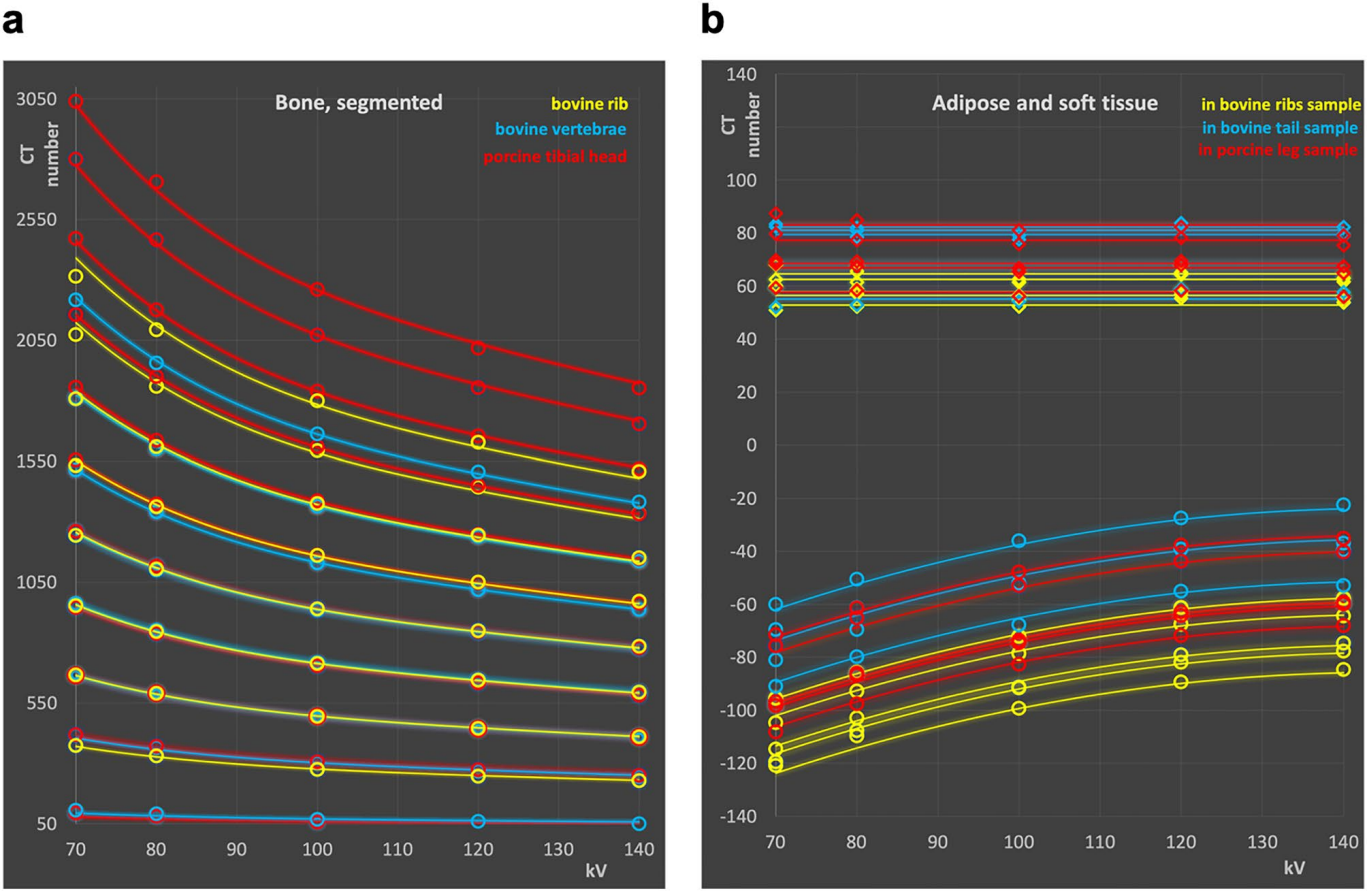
CT numbers from segmented bone samples (a), and soft tissues (b). Diamonds represent soft tissue (mainly muscular tissue), and circles adipose tissue. (a) and (b): Symbols: measured points, lines: model equation.
